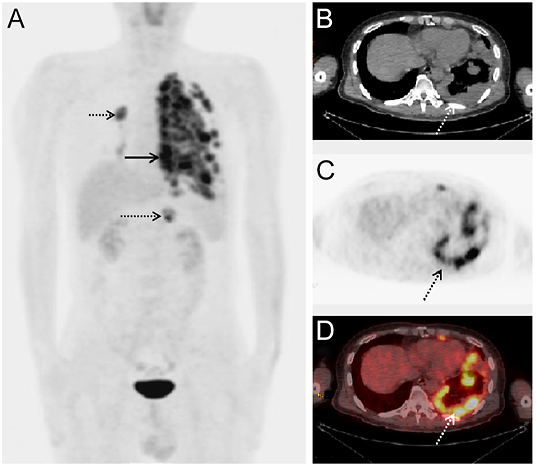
Representative case with PD. The patient was a 47-year-old man (case 11) who underwent total thyroidectomy with radical left neck dissection for PTC and was referred for ^131^I treatment of the postsurgical thyroid remnant and metastases. A ^131^I-WBS performed 5 days after oral administration of 7.4 GBq ^131^I did not show intense uptake. However, the serum Tg level increased to >25,000.00 ng/mL. Therefore, a ^18^F-FDG-PET/CT was performed to evaluate non-^131^I-avid lesions. The maximum intensity projection image (A) showed intense ^18^F-FDG uptake in lesions in the right chest (A, black short-dotted arrow) that were compatible with those in the left chest (A, black solid arrow) and abdomen (A, black long-dotted arrow). The increased activity seen on axial CT (B), PET (C) and fused PET/CT (D) scans indicated that ^18^F-FDG uptake was located in the left pleural region (B, C, D, dotted arrow), indicating PM. Although the patient is still alive, his disease has progressed and his Tg has remained >25,000.00 ng/mL persistently in the TSH suppressed status.
